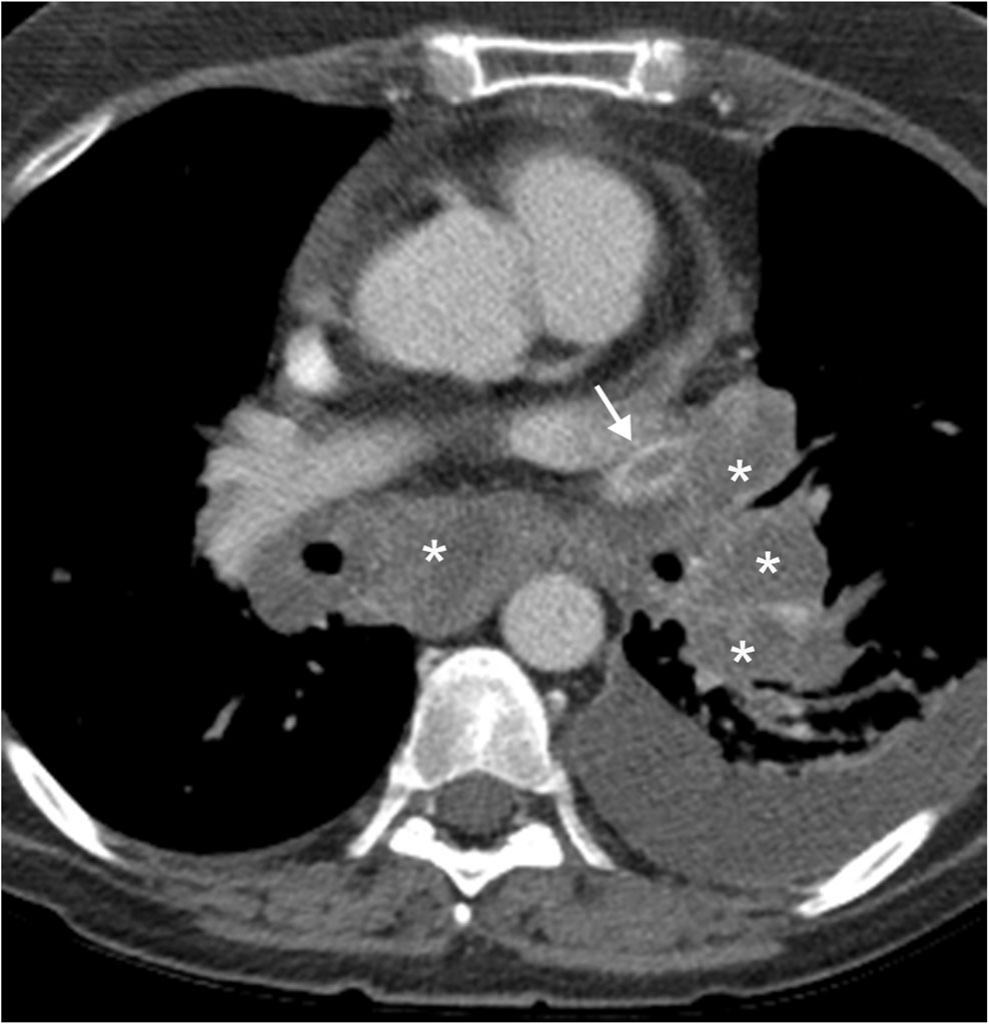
Pulmonary venous thrombus. Axial contrast-enhanced CT image in a patient with non-small cell lung cancer demonstrating a filling defect within the left superior pulmonary vein (*white arrow*). Note extensive lymphadenopathy within the left hila and mediastinum (*) from metastatic involvement
